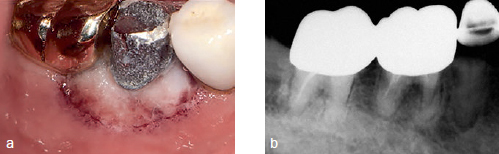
Clinical photograph and periapical view. a: Grey and fibrotic gingiva with well-delineated sloughing border are observed; b: alveolar bone resorption is shown.
